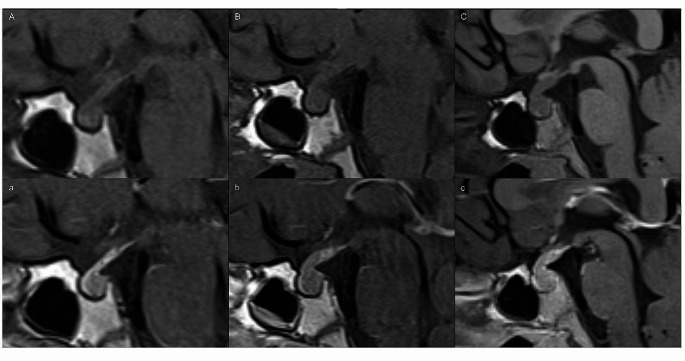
A case of a prolonged diagnosis. The patient experienced polydipsia and headaches for several months. The initial MRI examination revealed a loss of the posterior pituitary bright spot on a sagittal T1WI without contrast (A), and after gadolinium application (a), a tumor was found in the posterior pituitary gland.  T1-weighted images in (B, without contrast) and (b, with contrast) showed tumor growth and progressive pituitary stalk thickening 12 months later. Preoperative planning (C, c) did not occur until 20 months after the first MRI
